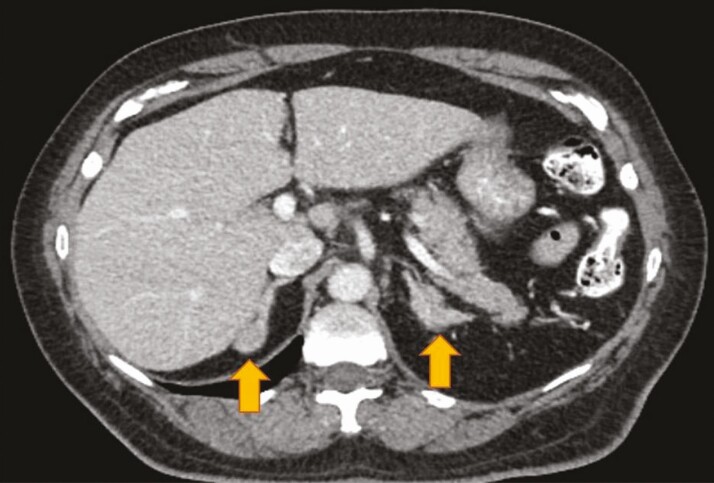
Cross-sectional abdominal computed tomography shows bilateral adrenal nodular hyperplasia (arrows) in Patient 1 with simple virilizing congenital adrenal hyperplasia.
